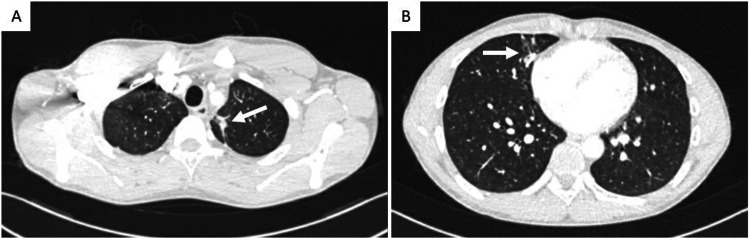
CT revealing residual lung densifications in the left upper lobe and the inner segment of the middle lobe (white arrows) Axial view
